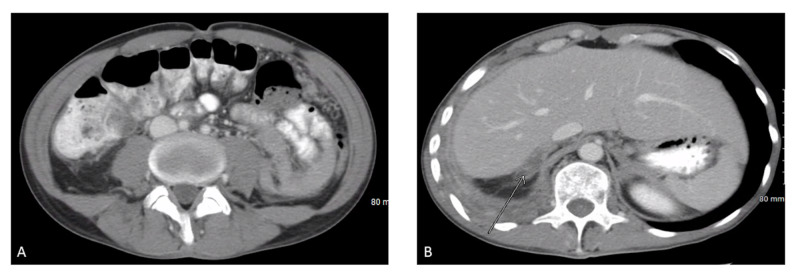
CT abdomen after two cycles of sunitinib treatment showing disease progression Figure A reveals peritoneal carcinomatosis and Figure B reveals pleural metastatic disease marked by an arrow.
